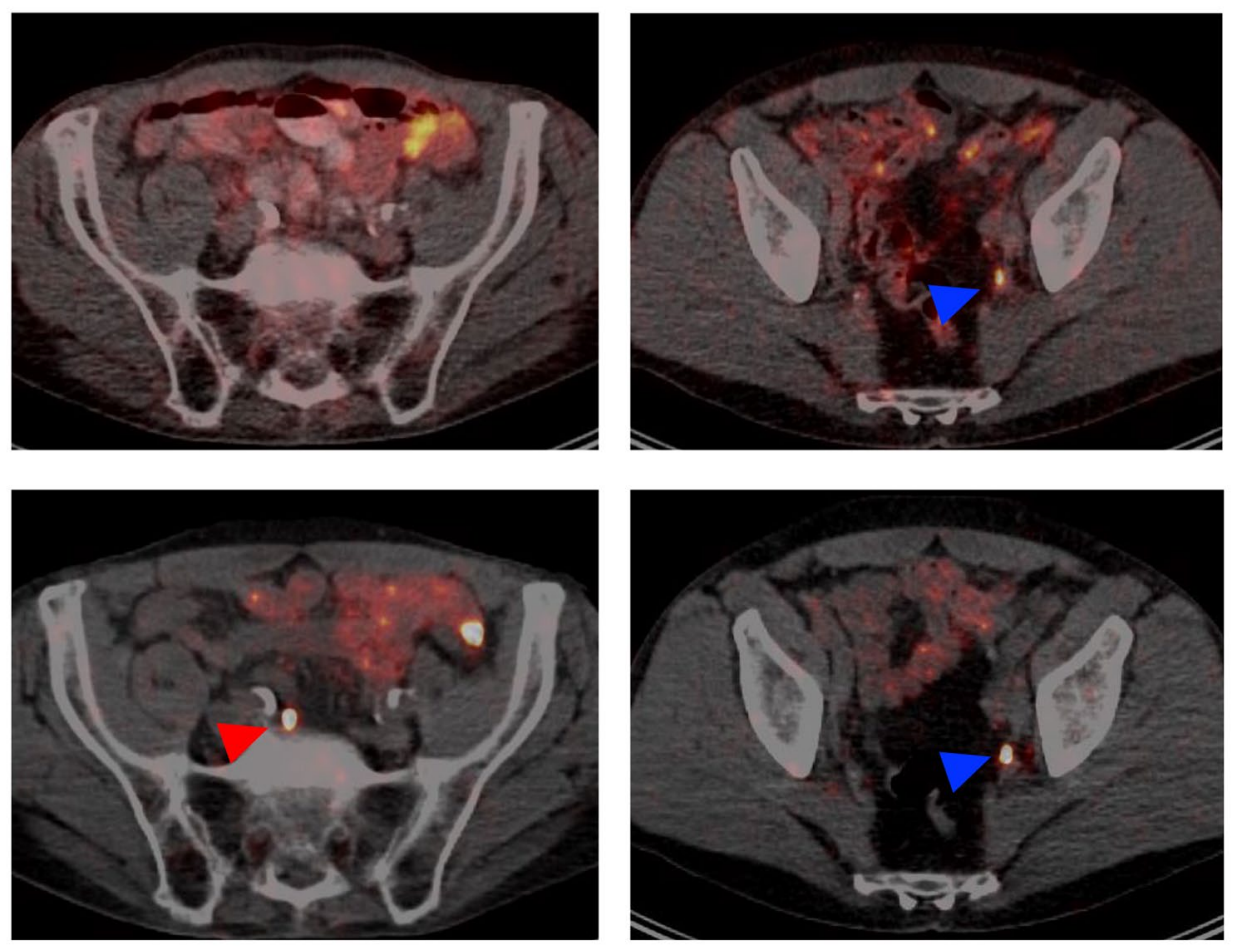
Representative transversal slice images, from two patients (one per column). The left-hand column shows a lesion (red arrow, bottom image) suspicious for lymph node metastasis of prostate cancer that was detected by [^89^Zr]Zr-PSMA-617 PET/CT (48-hr scan shown here) but not [^68^Ga]Ga-PSMA-11 PET/CT (top image). As exemplified in the right-hand column, all lesions detected on [^68^Ga]Ga-PSMA-11 PET/CT also were detected on [^89^Zr]Zr-PSMA-617 PET/CT (lesions indicated by blue arrows)
